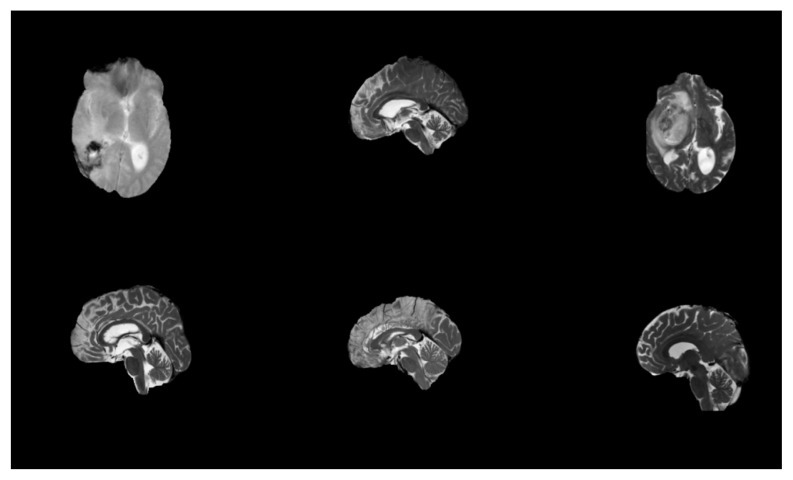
Original MRI scans from the dataset.
